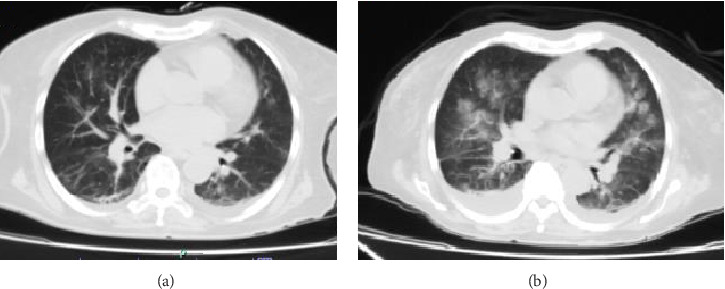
Chest computed tomography scan. The figure shows multiple perihilar infiltrates bilaterally, along with evidence of a predominantly right-sided pleural effusion (a) and bilateral perihilar alveolar compromise and bilateral basal pleural effusion, with an infectious component (b). Source: patient's medical chart, 2024.
